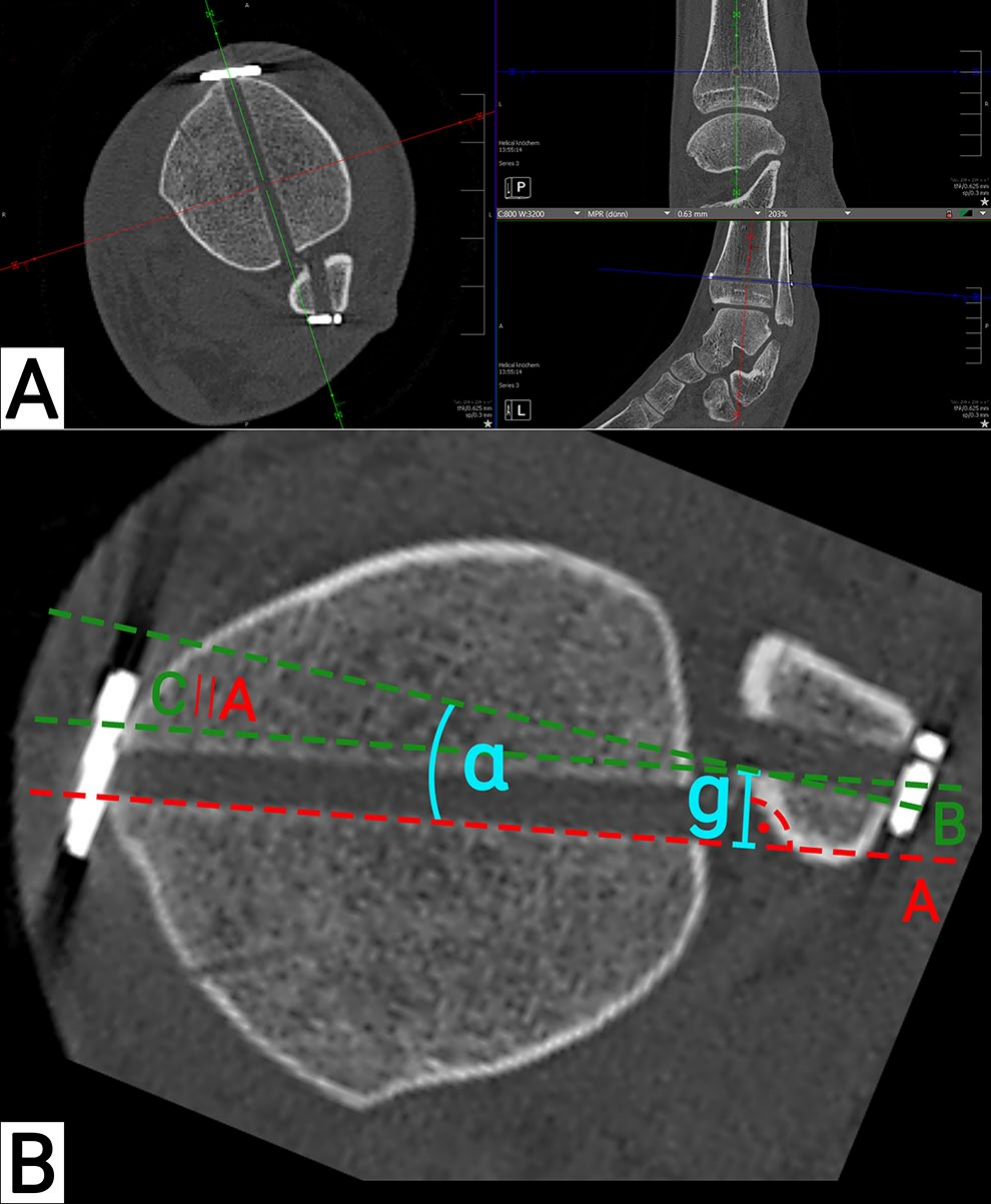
Suture button drilling tunnel measurements. (A) Computed tomographic
